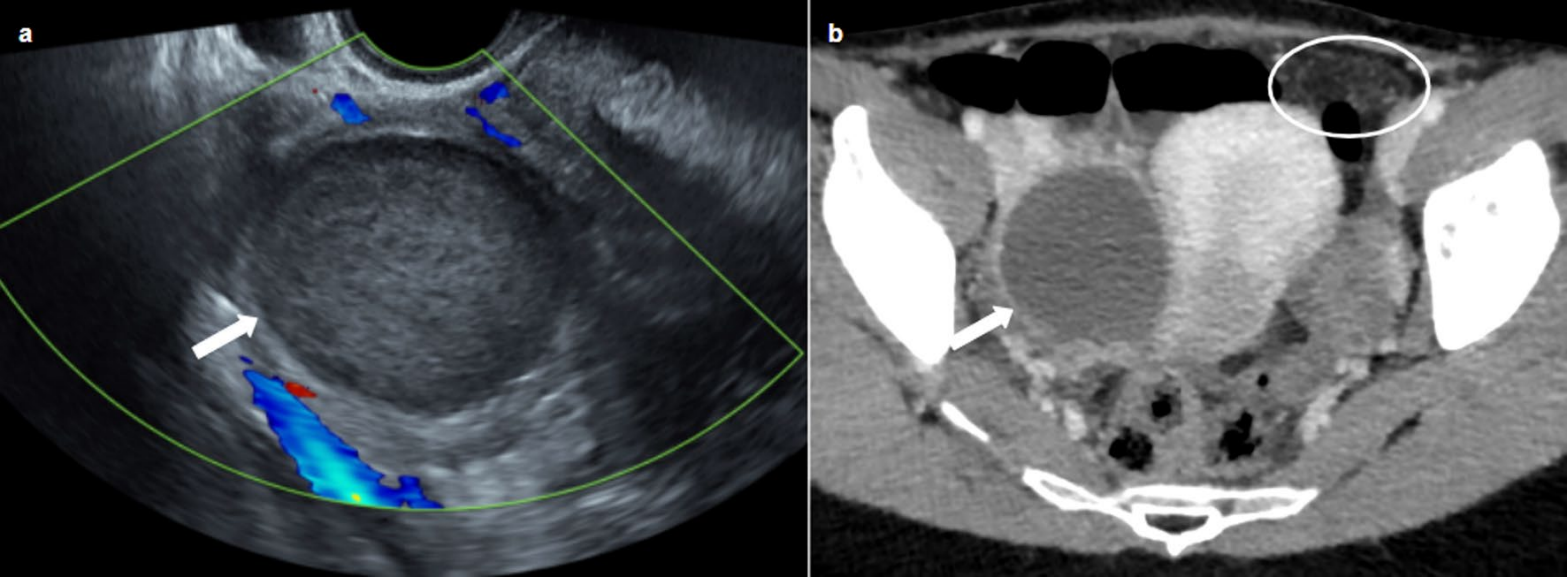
35-year-old female presenting with pelvic pain and elevated C-reactive protein (CRP). Transvaginal ultrasonography (a) showing a typical endometrioma of the right ovary: unilocular cyst with diffuse homogeneous ground-glass echoes. CT (b) showing a nonspecific cystic mass with uniformly low attenuation content (arrow), associated with pelvic fat stranding (circle)
